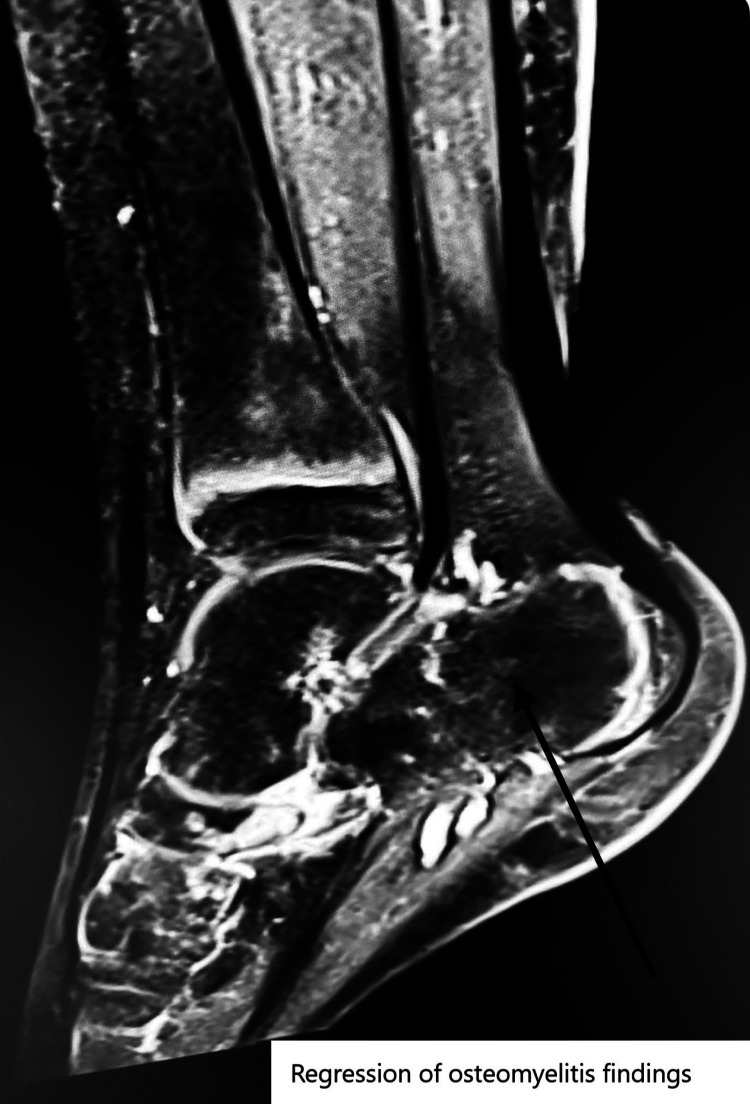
Regressive osteomyelitis findings on magnetic resonance imaging (arrow).
